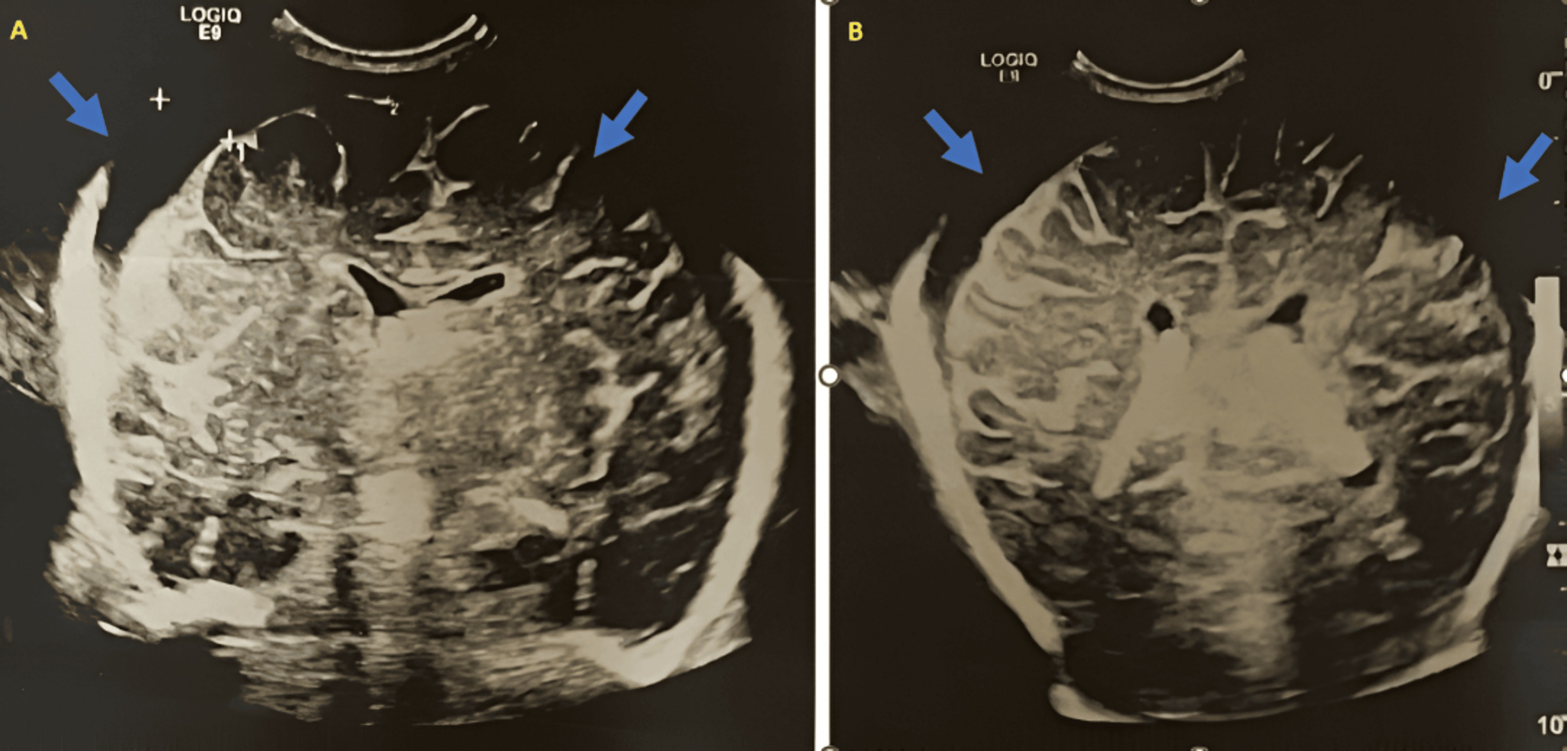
Head ultrasound showing subdural effusion (A, B) Coronal gray-scale head ultrasound images showing anechoic subdural fluid collections along the bilateral frontoparietal convexities, measuring 10 × 6 mm (blue arrows).
